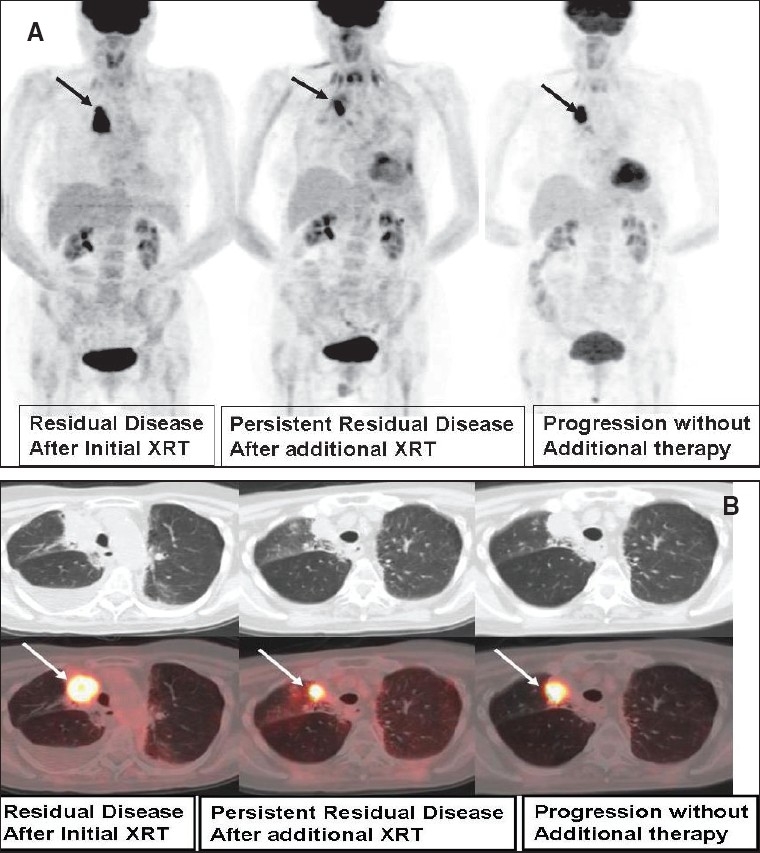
PET studies of a 79-year-old lady with a remote history of right lung cancer, with MIP (A) and axial (B) CT scan (top row) and fused PET/CT (bottom row) images at the level of the right upper lobe mass from three consecutive PET scans. Initial study after radiotherapy (left) shows an intensely FDG-avid right upper lung mass, proven to be recurrent neoplasm. A repeat PET study (middle) 4 months after completing concurrent chemoradiation therapy reveals incomplete response with small-to-moderate amount of intense residual disease. Since post-radiation therapy changes can last and remain intense for a considerable interval of time, the patient was observed without additional therapy. A follow-up PET study (right) 3 months later (7 months after completing chemoradiation) reveals disease progression
